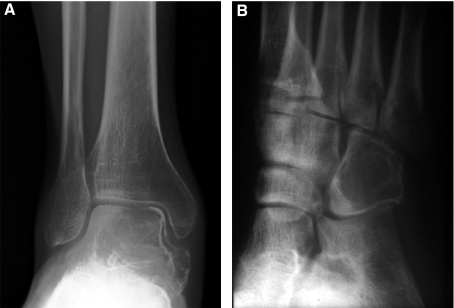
Radiograph of GCT involving the talus (A) and cuboid (B). As in Figure 1, both lesions are purely lytic with partially sclerotic rim, although the expansion of bone is not prominent. The lesions appear eccentric.
